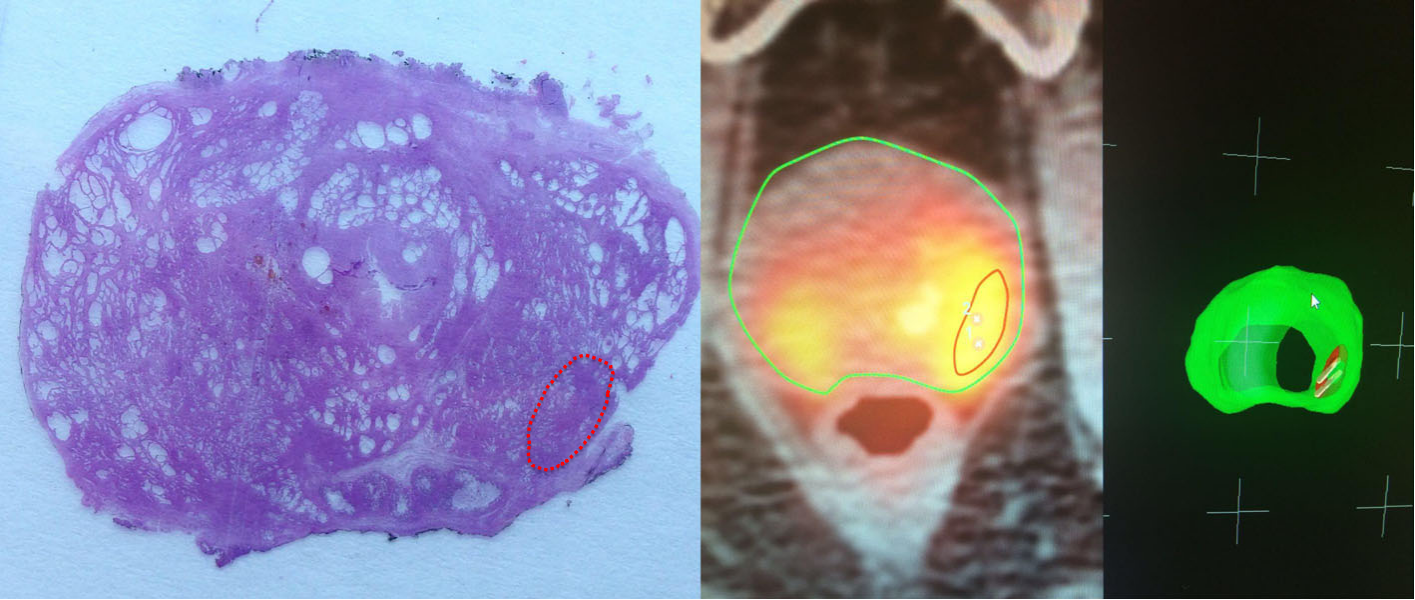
Left: Whole gland pathological examination. Red dot line indicates the PCa index lesion. Centre: Axial PET-CT/TRUS fusion imaging. Green line is the contour of whole gland and red line the ROI. Right: 3D imaging with biopsy samples (red cylinder)
